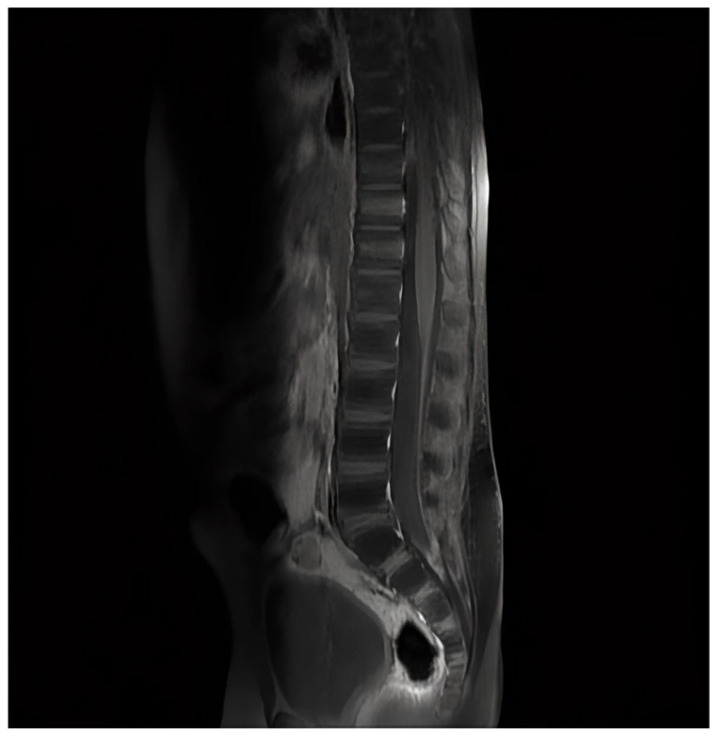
Follow-up Magnetic Resonance Imaging shows complete resolution of the previously noted meningeal enhancement.
